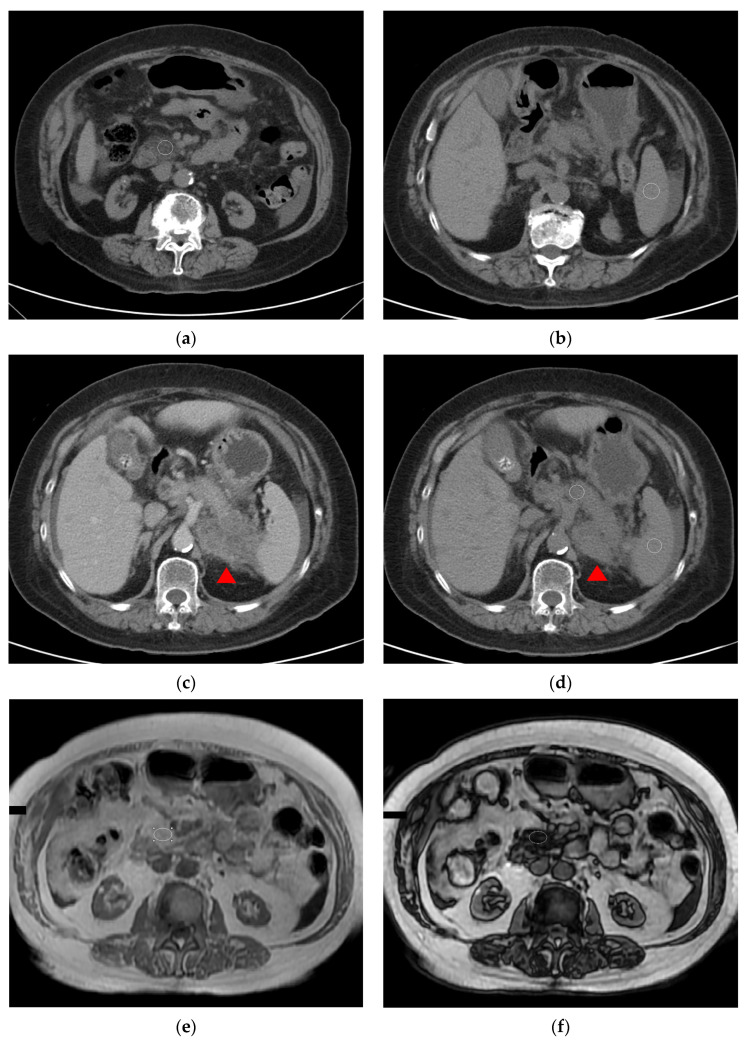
(a) Example of a patient with pancreas cancer and pancreatic steatosis in a non-contrast-enhanced CT scan (the main tumor site was located at the pancreas tail and was not shown), with measurement at the pancreas about average at −14 HU in the circular area. (b) Example of a patient with pancreas cancer and pancreatic steatosis in a non-contrast-enhanced CT scan (the main tumor site was located at the pancreas tail and was not shown), with measurement at the spleen about average at 44 HU in the circular area. (c) This image showed pancreatic cancer in the pancreatic tail (red triangle) on the contrast-enhanced CT scan. (d) This image showed the same level on the non-contrast-enhanced CT scan compared with Figure 1c. The circular area on the pancreas avoids the tumor site (red triangle) and reveals an average HU of about 23, and the circular area on the spleen reveals an average HU of about 44. (e) Example of a patient with pancreas cancer and pancreatic steatosis in an in-phase MRI (the main tumor site was located at the pancreas tail and was not shown), with the measurement at the pancreas about average at 859 in the circular area. (f) Example of a patient with pancreas cancer and pancreatic steatosis in an out-phase MRI (the main tumor site was located at the pancreas tail and was not shown), with measurement at the pancreas about average at 153 in the circular area.
